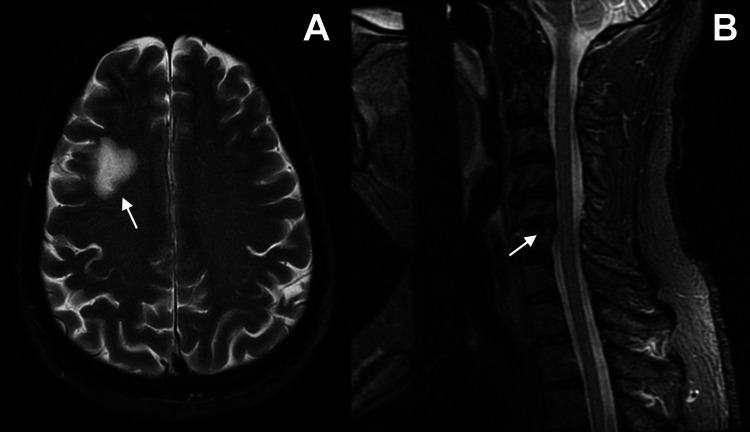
MRI of the patient’s head and neck. MR images of our patient’s head and neck obtained after the second clavicle surgery. (A) Axial T2 image showing a right frontal lobe lesion, likely related to a prior motorcycle accident, and (B) sagittal STIR image showing moderate degenerative disc disease at the C5-C6 level, causing moderate bilateral foraminal stenosis and mild central canal stenosis. As discussed below, it was initially hypothesized that these lesions might have contributed to a double- or triple-crush syndrome leading to the patient’s neurological deficits after the revision/final clavicle surgery. STIR: Short Tau Inversion Recovery.
